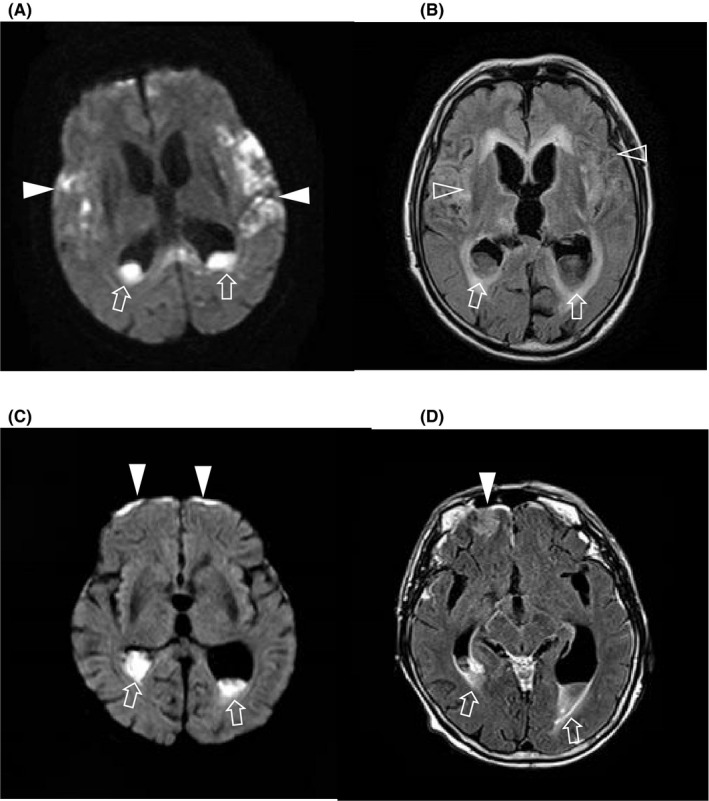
Brain MRI diffusion‐weighted image (A) and FLAIR image (B) in case 10 presenting with subarachnoid abscess (arrowheads) and ventriculitis (arrows) on hospital day 12. Brain MRI diffusion‐weighted image (C) and FLAIR image (D) in case 9 presenting with subarachnoid abscess (arrowheads) and ventriculitis (arrows) on hospital day 29
